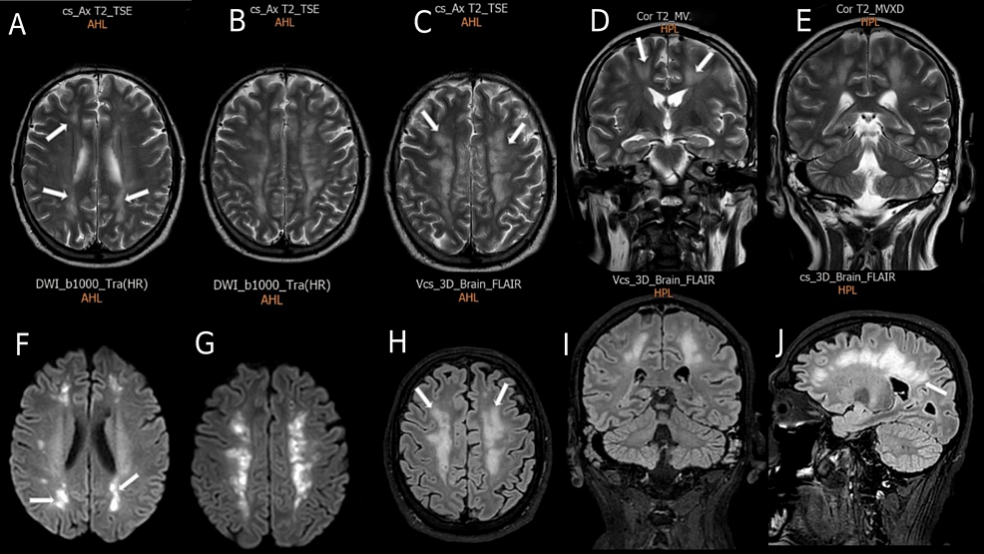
First MRI of the brain showing multiple bilateral periventricular and semiovale punctate and oval lesions (white arrows) with surrounding edema that show hyperintensity on T2W images (A, B, C, D, E) and FLAIR images (H, I, J) with diffusion restriction (F, G). T2W = T2-weighted; FLAIR = fluid-attenuated inversion recovery
